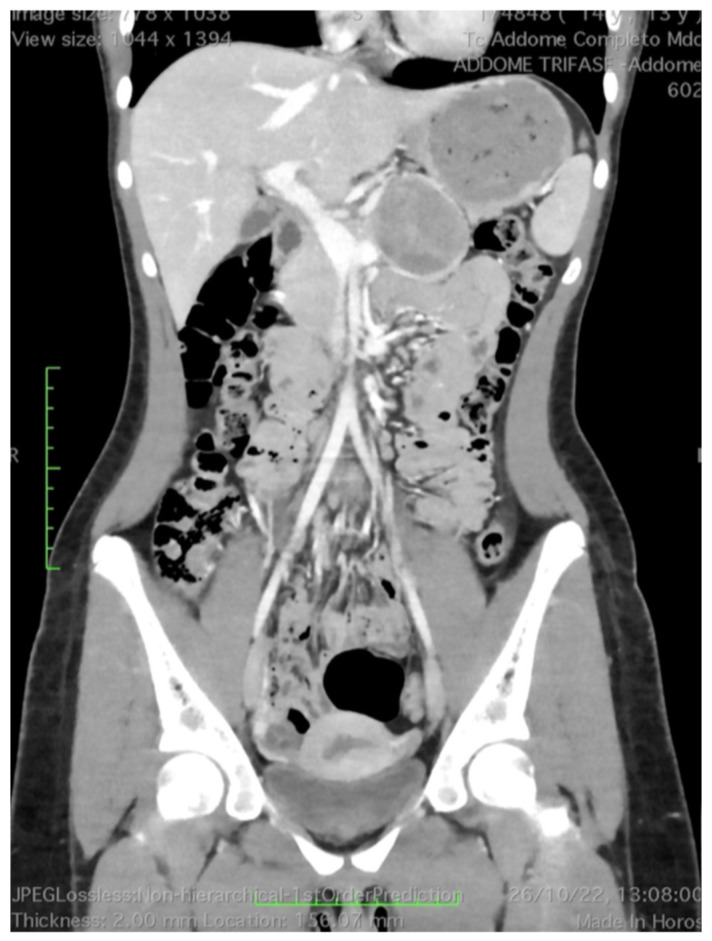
Coronal contrast-enhanced CT scan of the abdomen (portal venous phase). A large, well-defined heterogeneous mass is located at the superior margin of the pancreatic neck and body, measuring approximately 5 × 4 × 5.5 cm. The lesion displays smooth borders and a mixed composition consisting of hypodense cystic areas and hyperdense solid components. The mass is positioned cranially to the splenic vein, which shows luminal narrowing due to extrinsic compression, and inferiorly to the splenic artery, which is displaced cranially. No signs of loss of the fat plane or irregular vessel wall contouring are observed. Green scales are measures of length indicated in cm.
